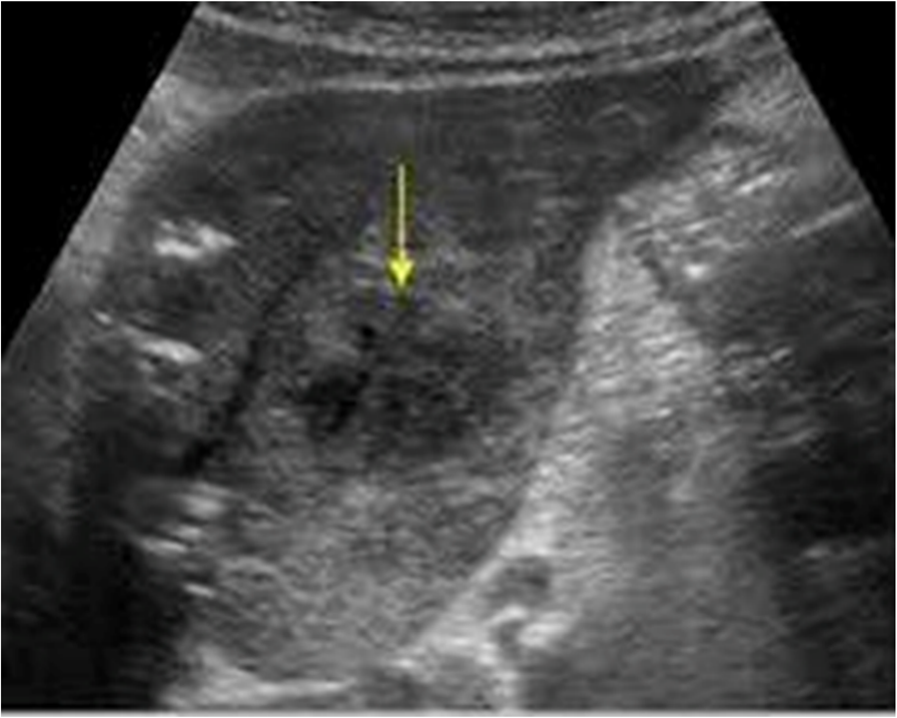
Abdominal ultrasound image of hepatic abscess (old). The arrow is pointing towards hepatic abscess
